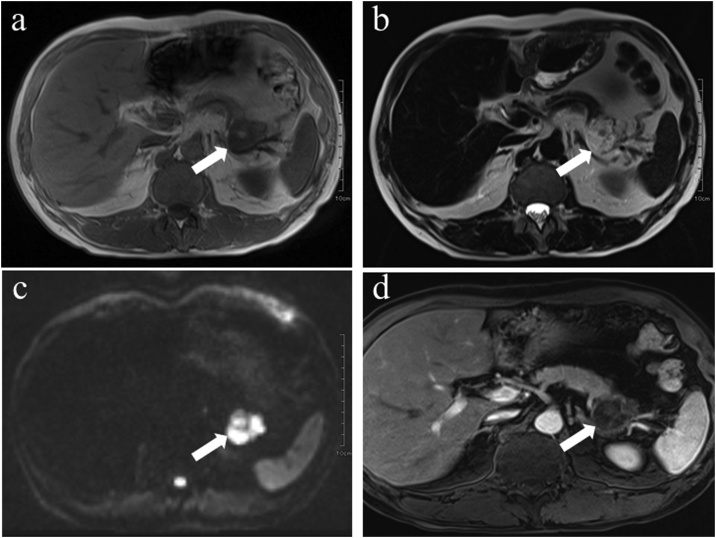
Magnetic resonance imaging (MRI): A. MRI demonstrated a multiple-ball-like lesion of low signal intensity on T1-weighted image (arrow). B. MRI showed the same lesion with high signal intensity on T2-weighted image (arrow). C. MRI showed high signal intensity in the central and iso-signal intensity in the peripheral portions of the cystic lesion on diffusion-weighted image (arrow). D. Enhanced MRI showed high signal intensity in the cystic wall and septa and low signal intensity of the cystic contents (arrow).
